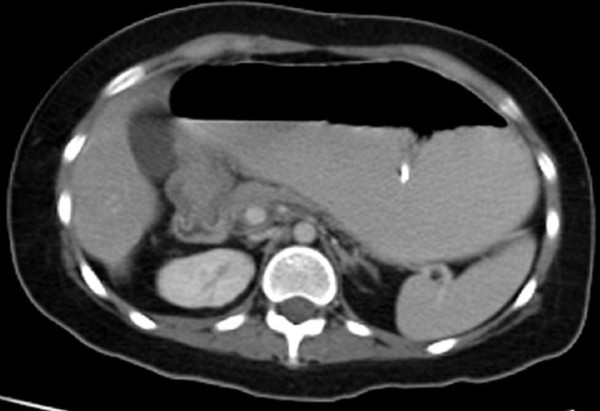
CT scan of the abdomen with oral contrast demonstrating markedly dilated stomach, abnormal thickening in the region of the antrum and pylorus of the stomach, and adjacent pancreas thinned due to compression from dilated stomach. There was no intra-abdominal adenopathy.
